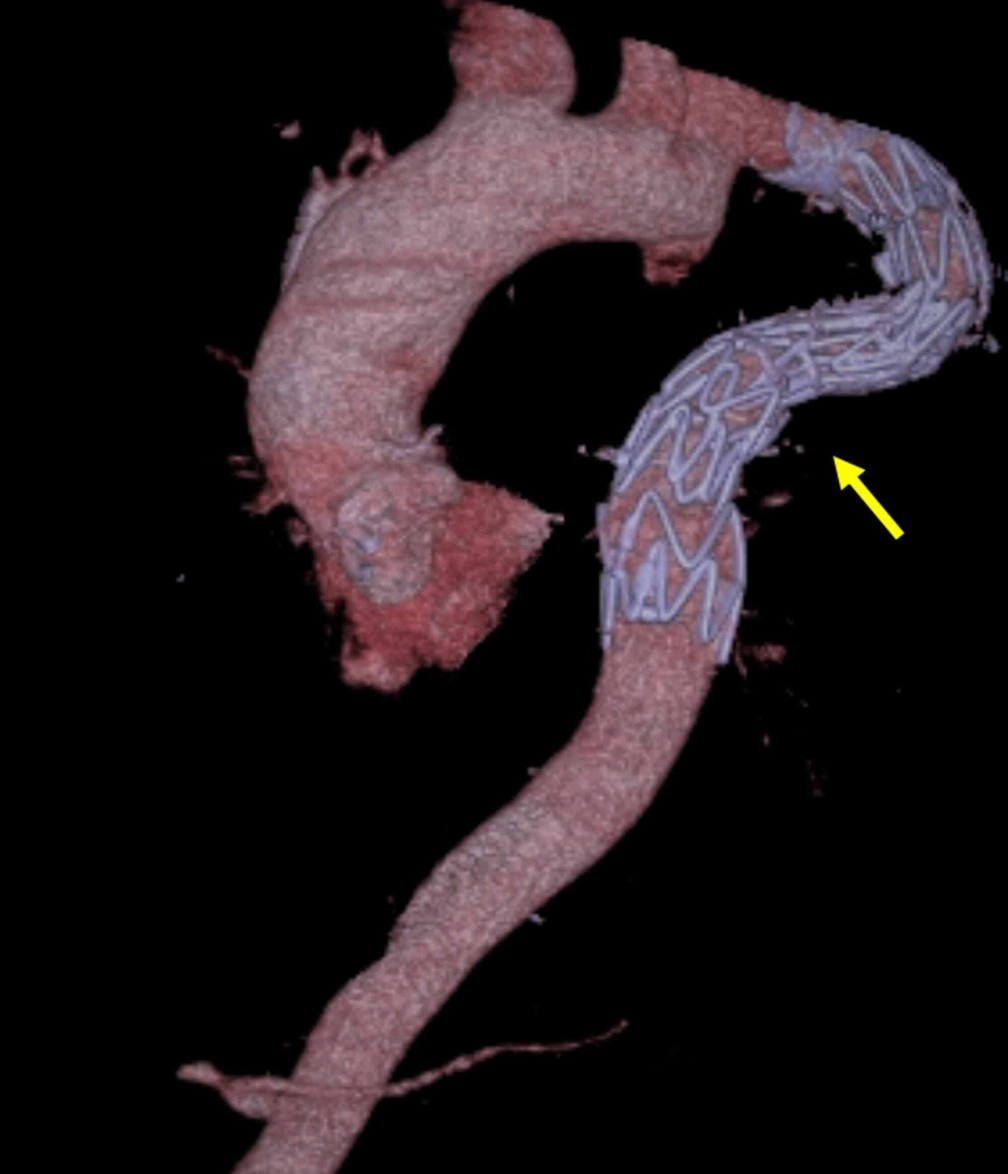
Computed tomography scan of the aorta revealing a patent stent graft with no endoleak
